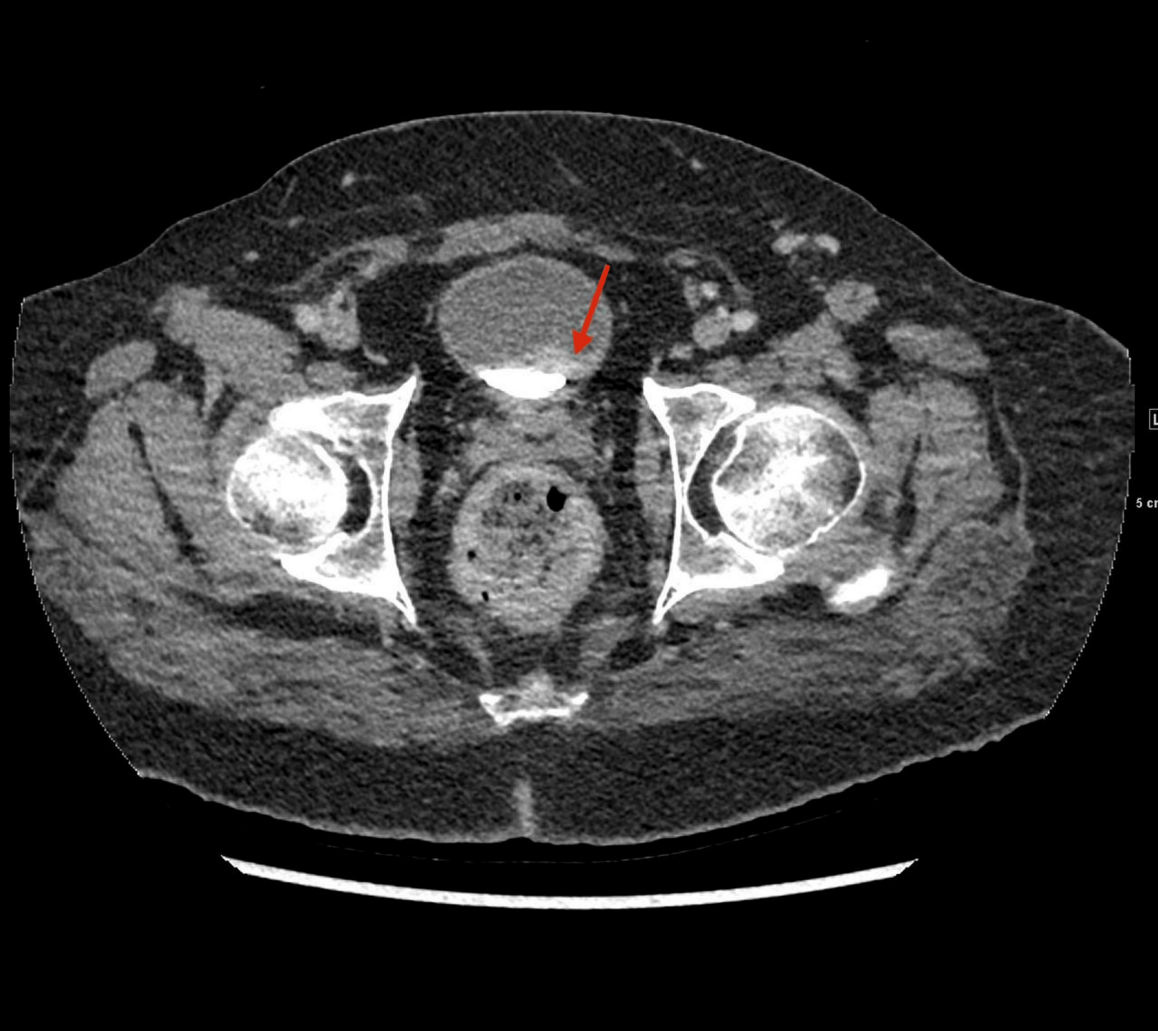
CT urography (axial view) showing a heterogeneous soft-tissue mass (arrow) arising from the left lateral bladder wall. The lesion projects into the bladder lumen as a filling defect with an irregular, enhancing contour, consistent with a bladder tumor. Abnormal finding: irregular intravesical mass. Normal findings: surrounding pelvic structures (prostate and pelvic bones) are intact with no spread outside the bladder.
